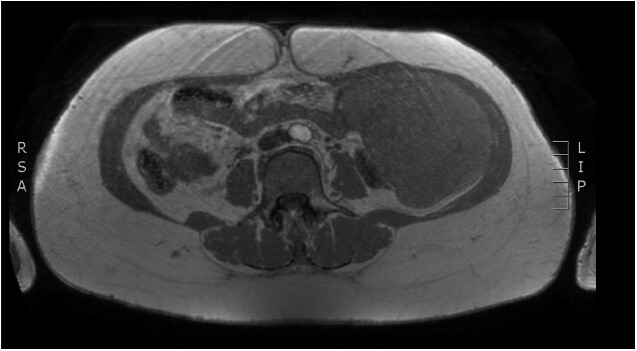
Preoperative MRI—saggital plane. T1 weighted image.
